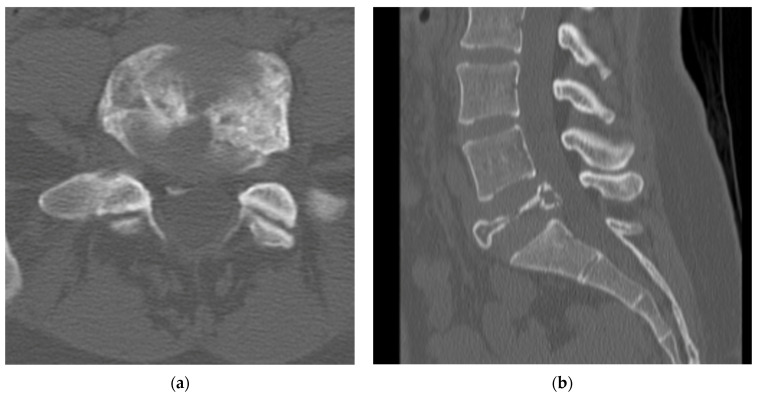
(a) L5 Giant Cell Tumor after 6 months of IV denosumab treatment. Axial cuts of L5 vertebral body show significant ossification of tumor with reestablishment of previously eroded cortices. (b) Sagittal cuts of L5 Giant Cell Tumor after 6 months of IV denosumab therapy show increased loss in vertebral height from previous CT scan; however, cortical margins are reestablished. There appears to be mild retropulsion of L5 vertebral body.
